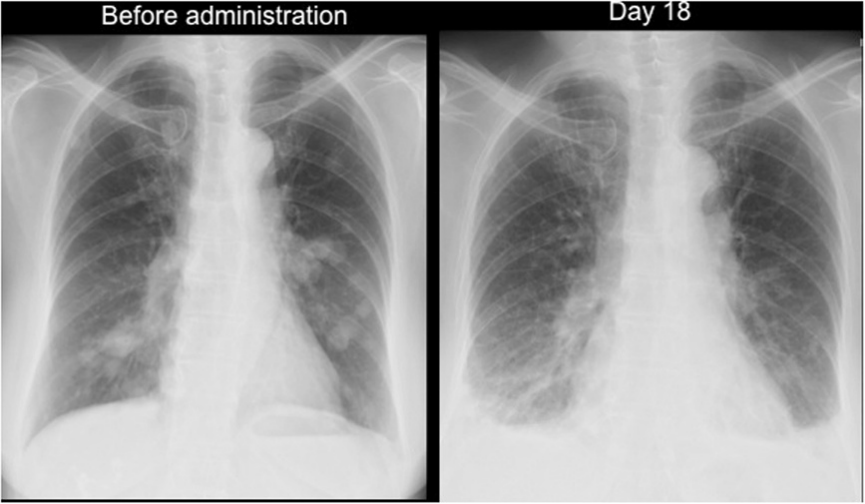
Chest X-ray images. Chest X-ray images before and on day 18 of sunitinib administration revealed bilateral multiple lung nodules and bilateral pleural effusion, respectively.
